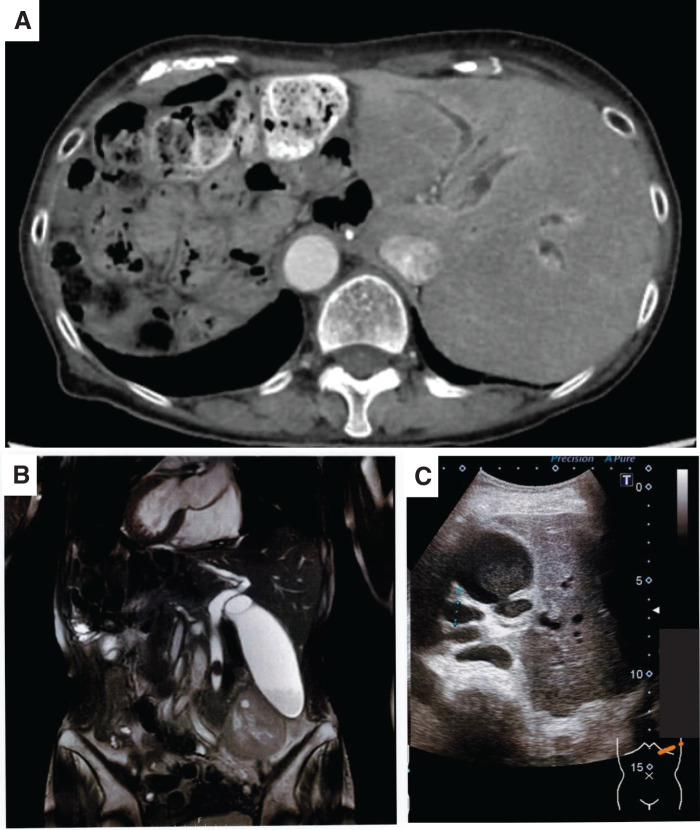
Preoperative imaging findings. (A) Contrast-enhanced CT showing mirror-image positioning of abdominal organs consistent with situs inversus totalis. (B) Magnetic resonance cholangiopancreatography revealing an 18-mm stone within the common bile duct, accompanied by upstream biliary dilation. (C) Ultrasonography demonstrating choledocholithiasis with posterior acoustic shadowing.
